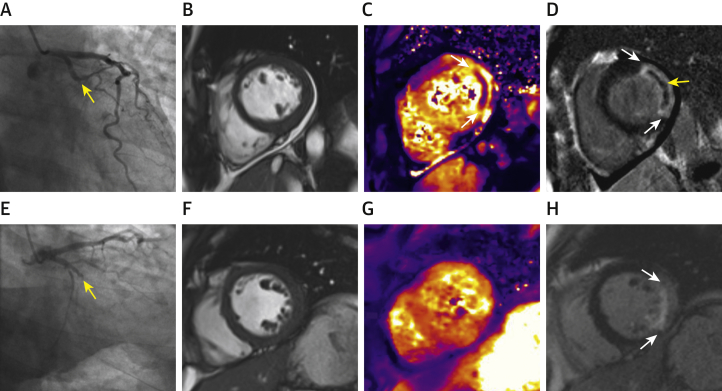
Smoking and Myocardial Hemorrhage After Acute ST-Segment Elevation Myocardial Infarction Current smoker (A to D) and nonsmoker (E to H) who underwent primary percutaneous coronary intervention (PCI) for lateral ST-segment elevation myocardial infarction. Both had TIMI (Thrombolysis in Myocardial Infarction) flow grade 3 post-PCI. Antithrombotic therapies, including aspirin, clopidogrel, and unfractionated heparin, were similar for both patients. Magnetic resonance imaging (MRI) was performed 2 days and 6 months later. (A to D) Imaging from a 58-year-old male current smoker. Symptom-to-balloon time was 8.5 h. Angiography revealed a proximally occluded circumflex coronary artery (yellow arrow, A). Two days later, myocardial hemorrhage was revealed by T2* mapping (white arrow, C). Late gadolinium enhancement revealed transmural infarction of the lateral left ventricular (LV) wall (white arrows, D) associated with microvascular obstruction (MVO) (yellow arrow, D). Baseline infarct size was 32.3%, LV ejection fraction 55%, and LV end-diastolic volume indexed to body surface area (LVEDVi) 83.8 ml/m^2^. Six months later, infarct size was 31.2% and LVEDVi 84.8ml/m^2^, consistent with adverse remodeling. (E to H) Imaging from a 48-year-old male nonsmoker. Symptom-to-balloon time was 9.3 h. Angiography revealed a proximally occluded circumflex coronary artery (yellow arrow, E). Two days later, there was no evidence of myocardial hemorrhage (G) or MVO within the infarct zone (H) on MRI. Baseline infarct size was 25.3%, LV ejection fraction 51%, and LVEDVi 78.4 ml/m^2^. Six months later, infarct size was 15.2% and LVEDVi 72.7 ml/m^2^.
